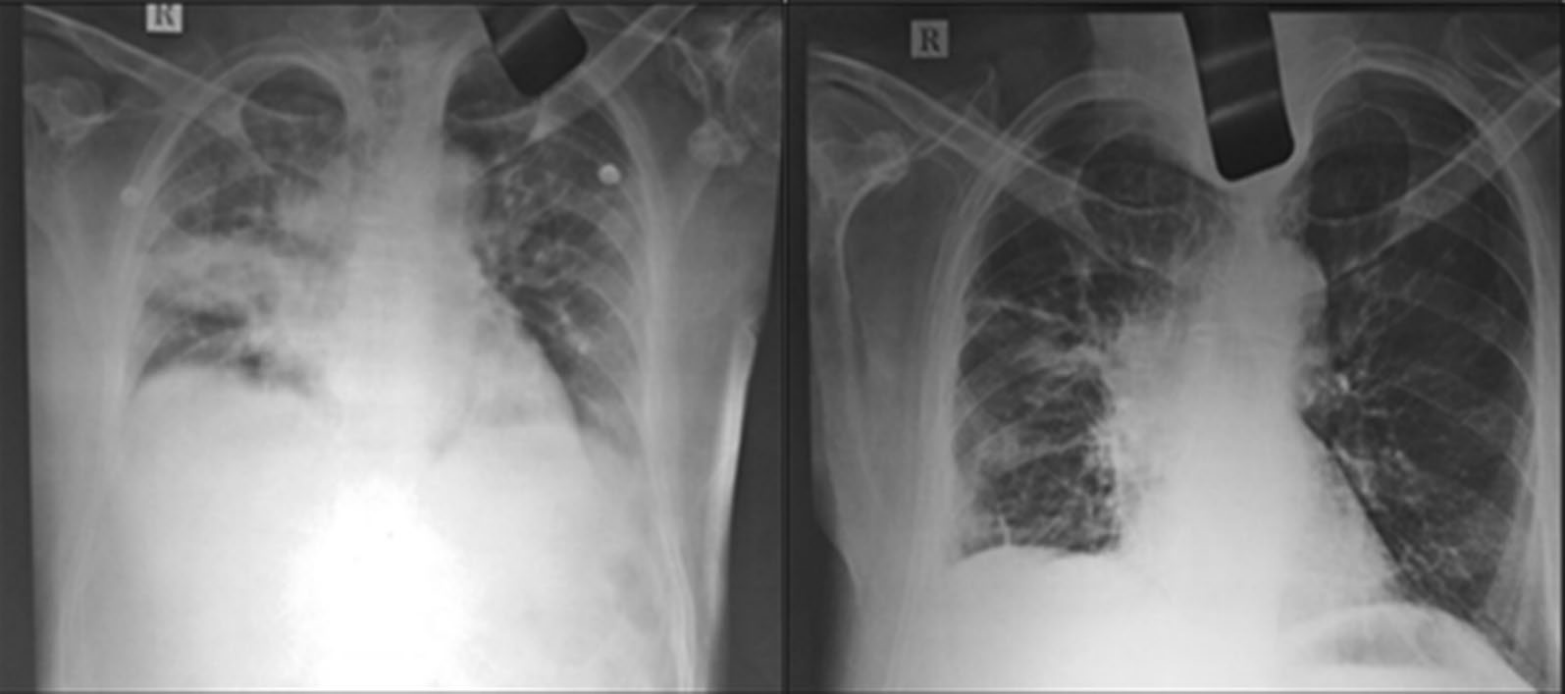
a X-ray of the lungs before treatment (Left Image) b X-ray of the lungs after treatment (Right image)
